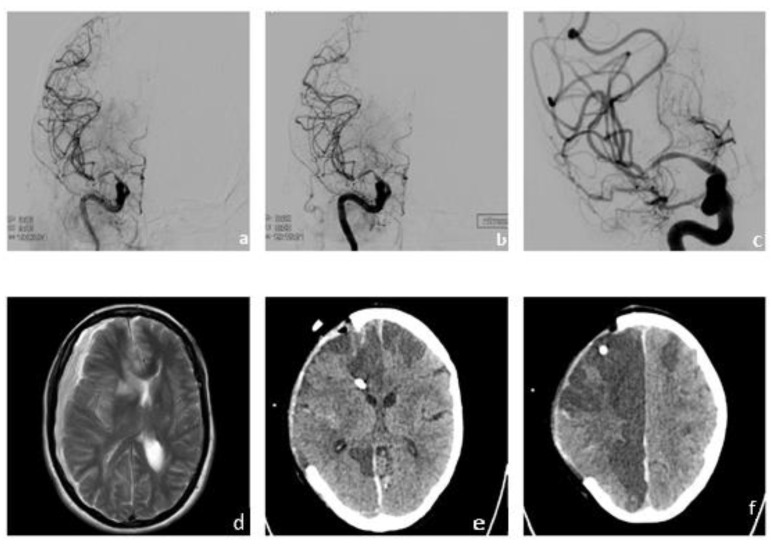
Follow-up imaging with DSA-series, MRI and CT imaging. DSA-series showing successful stent implantation in the right MCA with improved vessel diameter/perfusion (a–c). MRI and CT imaging demonstrating late-onset complications with severe infarct demarcation in both hemispheres and right hemispheric intracerebral hemorrhage as well as subdural hematoma requiring neurosurgical intervention and hemicraniectomy (d–f).
